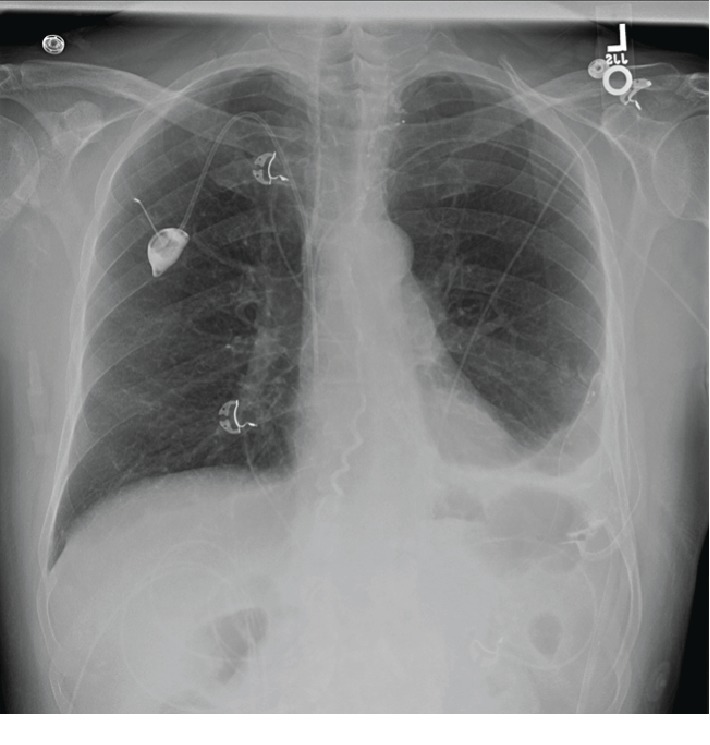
Chest X-ray after thoracic duct lymphangiogram using glue embolization, with resolving chylothorax in the left hemithorax.
